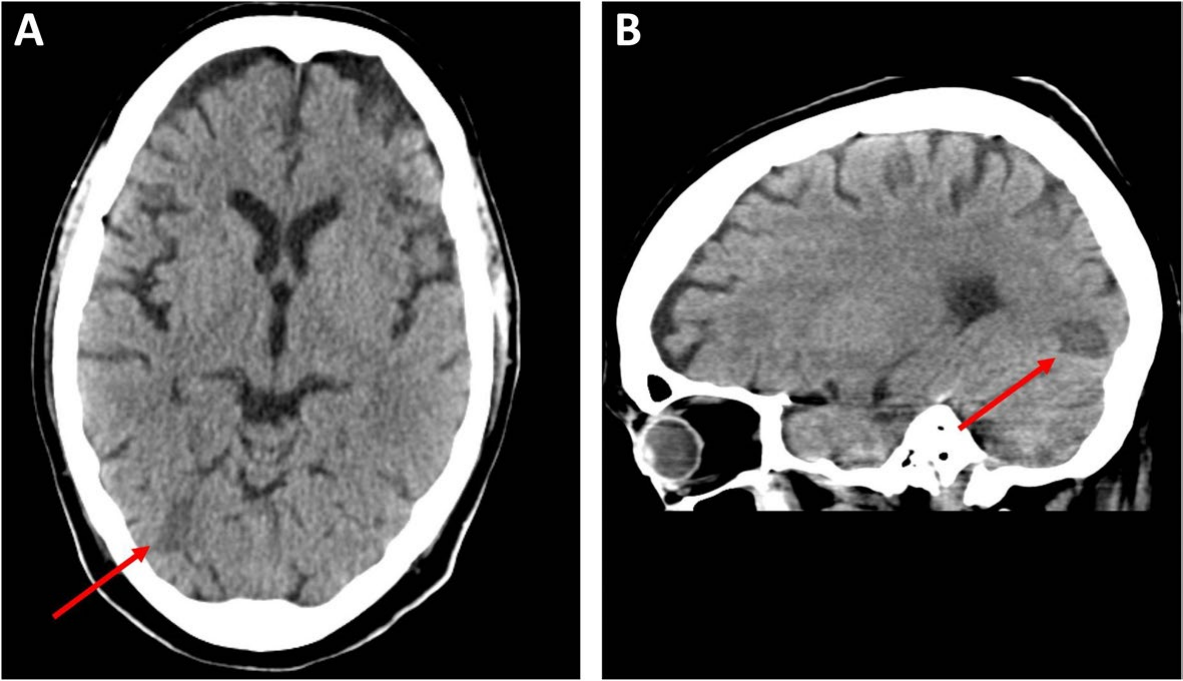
Computed tomography scan of the brain showing a small right sided posterior infarct, A transversal and B sagittal (red arrows)
